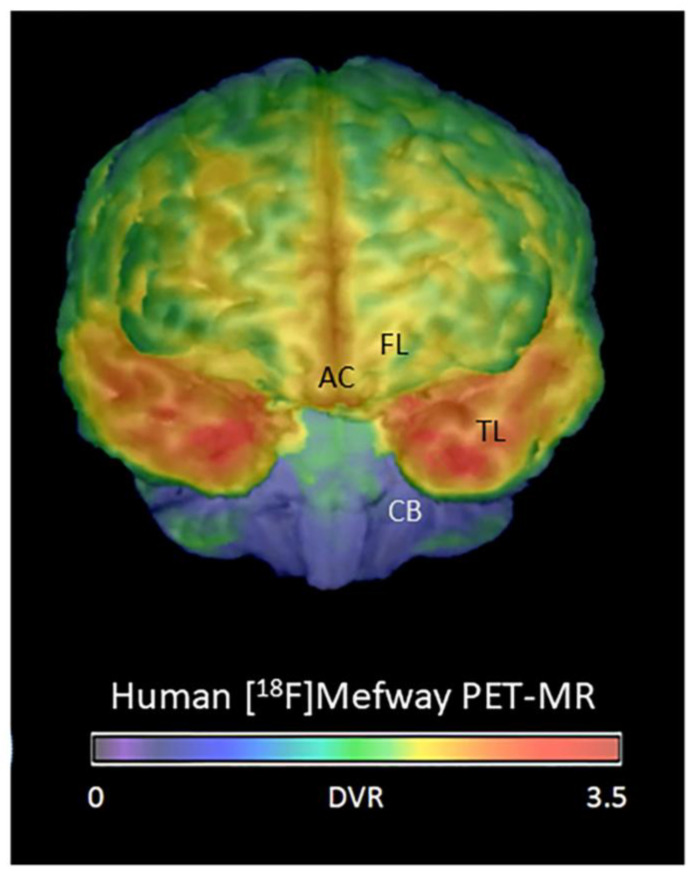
[^18^F]mefway for imaging human serotonin 5HT_1A_ receptors. Brain surface image of [^18^F]mefway PET-MRI from cognitively normal subject showing binding of [^18^F]mefway. High binding of [^18^F]mefway is seen in the temporal lobe (TL; red) which includes the hippocampus, moderate binding in frontal lobe (FL; yellow) and anterior cingulate (AC; yellow red) and least in cerebellum (CB; blue).
